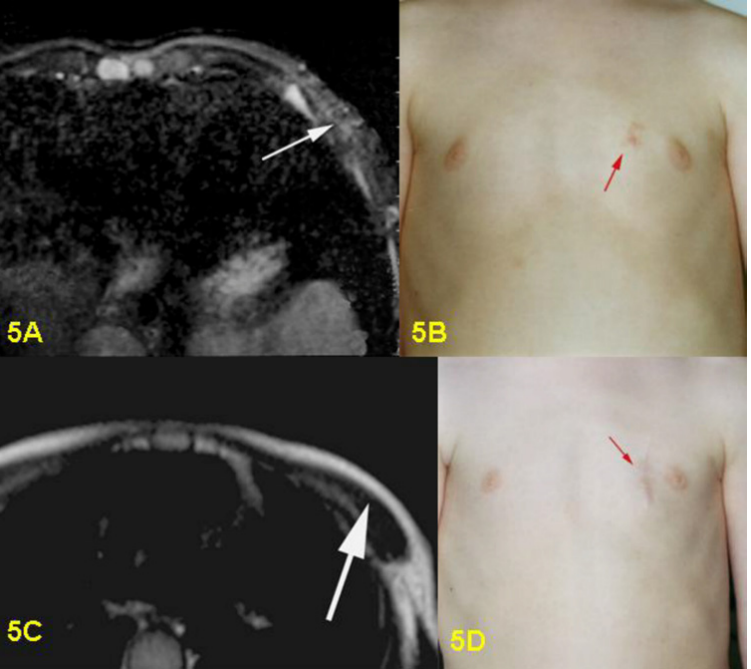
5A: Pre-surgical T2-weighted STIR-sequence, axial section; small nodular hyperintensive PNF in the cutis and subcutis on left ventral chest wall. 5B: Pre-surgical clinical view of PNF in left chest wall, parasternal. 5C: Post-surgical T2-weighted Turbo Spin Echo-sequence, axial section: tumor no longer visible, only adipose tissue visible in the subcutis. 5D: Post-surgical clinical view of scar.
